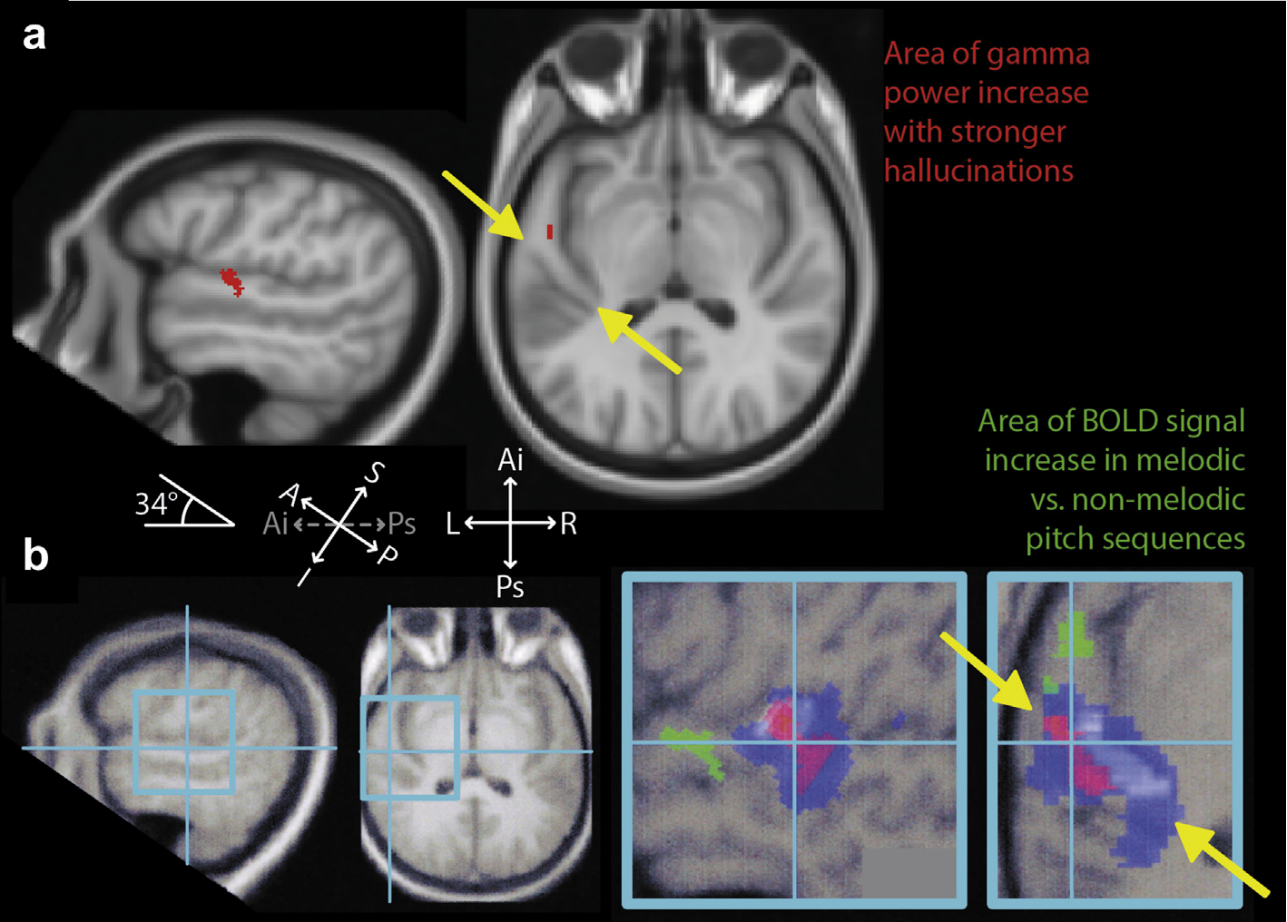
Gamma band (30–60 Hz) oscillatory power increases, in a cortical area specialised for processing pitch sequences (anterior superior temporal gyrus; aSTG), associated with increased (high *vs* low) hallucination intensity. Heschl's gyrus, containing core auditory cortex, runs from posteromedial to anterolateral (ends denoted by yellow arrows), and aSTG is located anterior to its anterolateral end. a: Areas of significant gamma power increase surviving whole-brain correction (red areas) displayed on saggital (left) and axial (middle) sections, of a standard template MRI scan, with a 34° tilt applied. b: For comparison purposes, the results from a single typical subject from (Patterson et al., 2002) are shown (right) in equivalent tilted saggital and axial sections. The two plots on the left show the positions in the brain of the two enlarged regions on the right. The area responding selectively to melody is shown in green, falling precisely within aSTG, while blue and red areas indicate areas responding to noise and to the pitch of single notes respectively related to Heschl's Gyrus (shown in white). Abbreviations: S = superior, I = inferior, A = anterior, P = posterior, Ai = antero-inferior, Ps = postero-superior.
